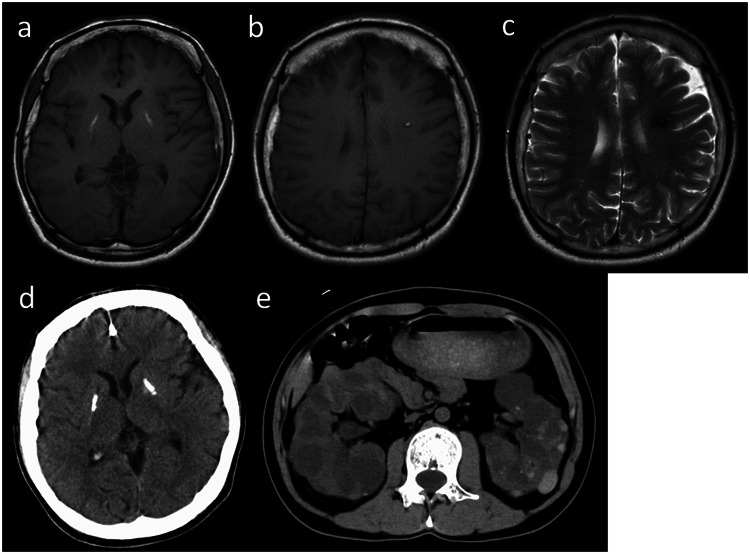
Imaging results of the patient. Brain magnetic resonance imaging (MRI) and head computed tomography (CT) were performed when the patient was 32 years old. a, b T1 weighted imaging (T1WI), c T2WI, d head CT, and e abdominal MRI.
